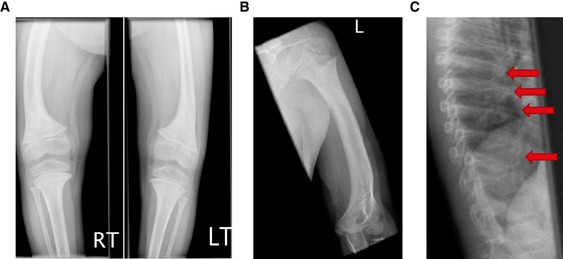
Radiographs demonstrate bowing deformity, short long bones, and vertebral fractures of the affected patient A–C(A) Bilateral bowing deformity and short femora and tibiae with atypical growth plates, (B) short and bowed humerus at age 4.5 years, and (C) vertebral fractures (arrows) on lateral imaging at age 9 years.
